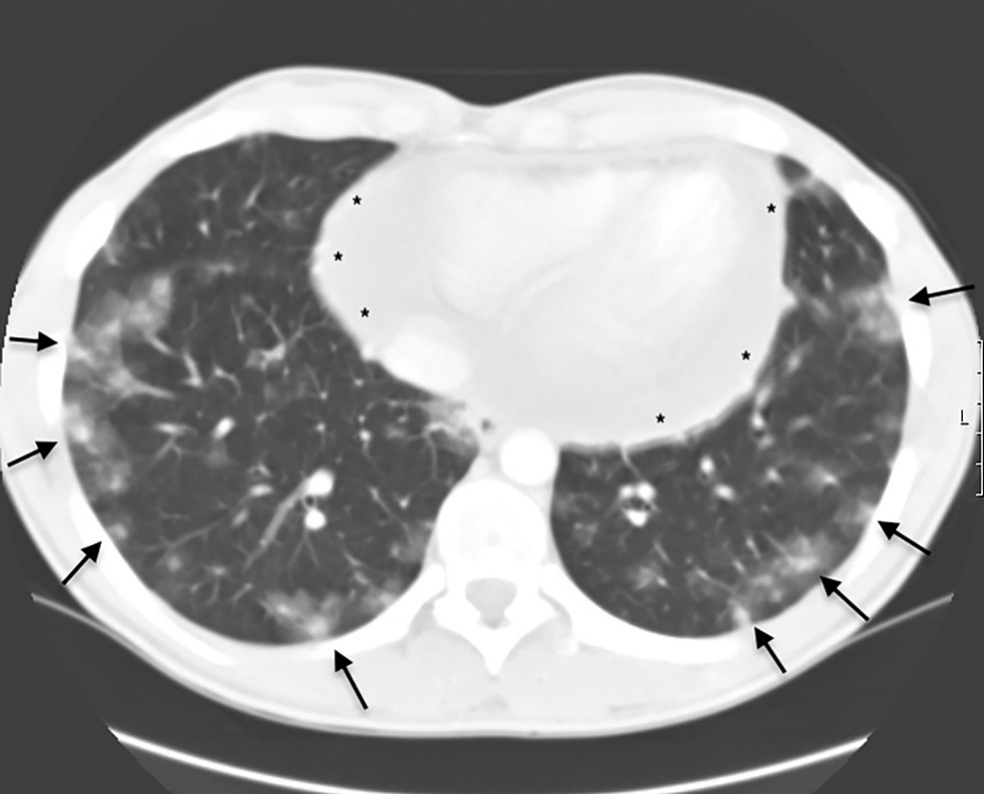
Thoracic CT scan. Thoracic CT demonstrated bilateral dispersed hypodense pulmonary nodes with peripheral halo, alveolar densification (arrows), and a large pericardial effusion (*).
